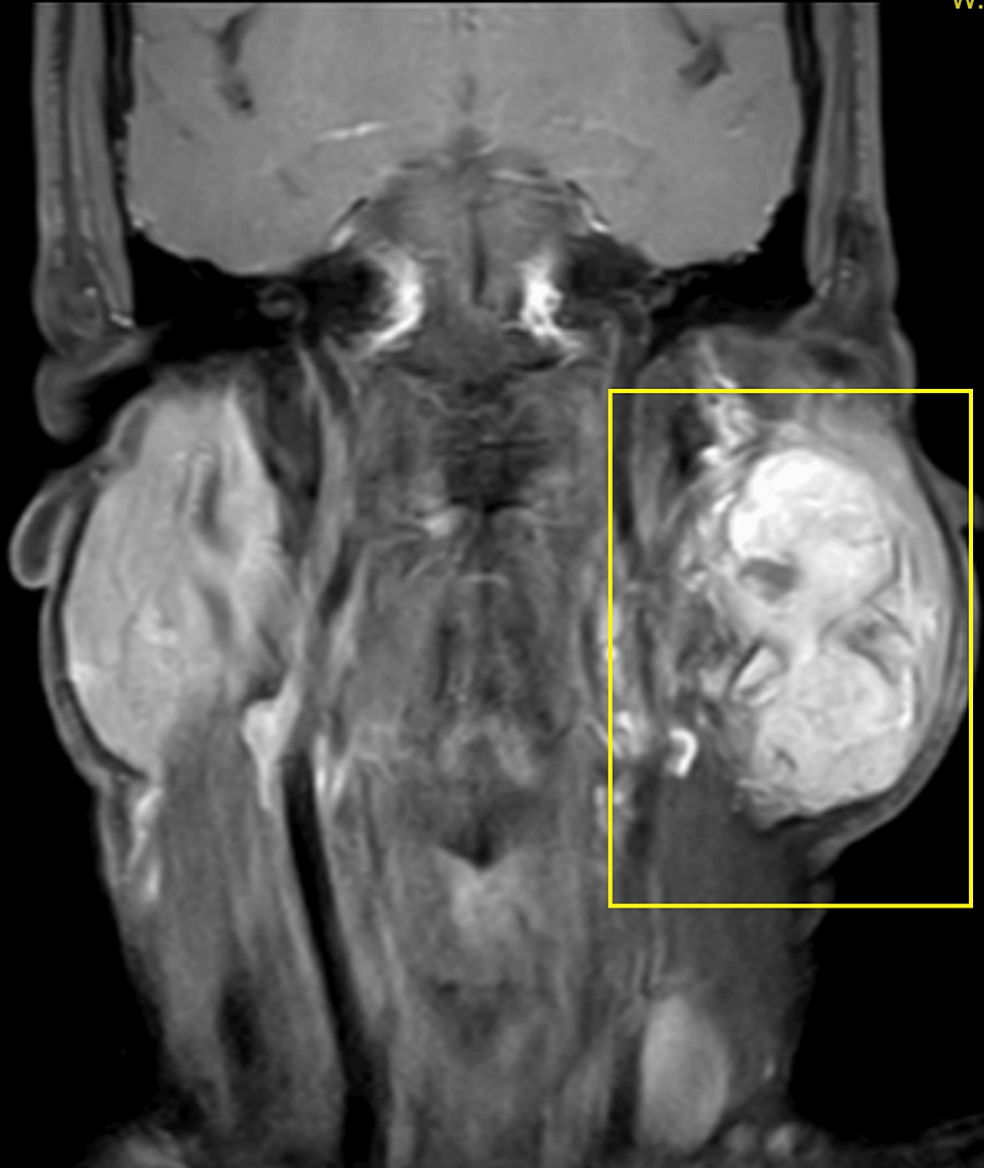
T1WI post-contrast coronal MRI image Highlighted area shows the physical presentation of parotid gland tumor T1WI, T1-weighted image
